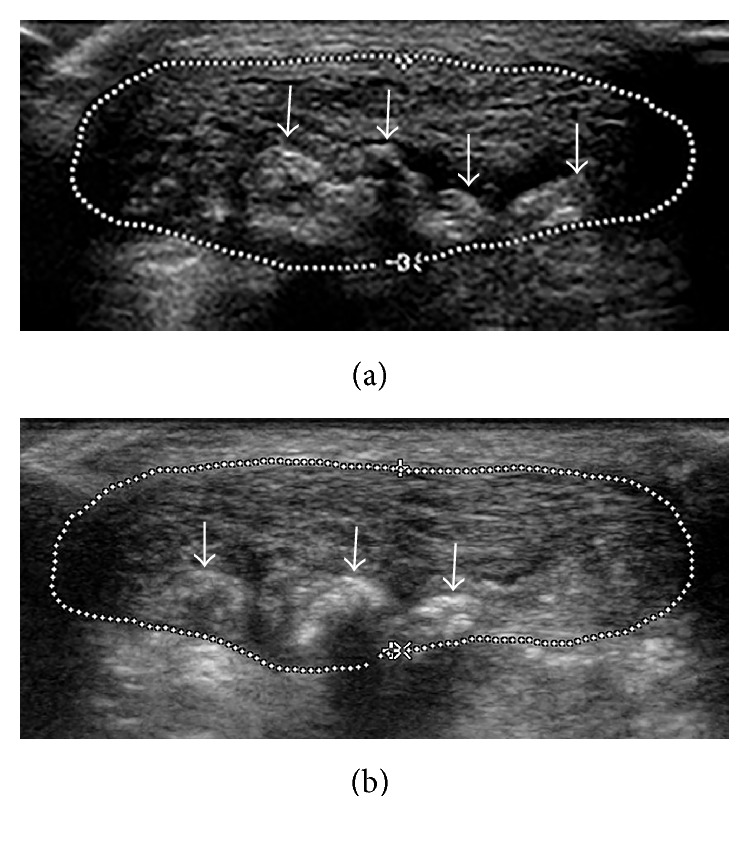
(a) Transverse sonogram shows plentiful calcific plaques (arrows) in the insertion of the Achilles tendon before therapy (outline of Achilles tendon traced by dotted line). (b) The calcific plaques reduced by the 4th week after therapy.
